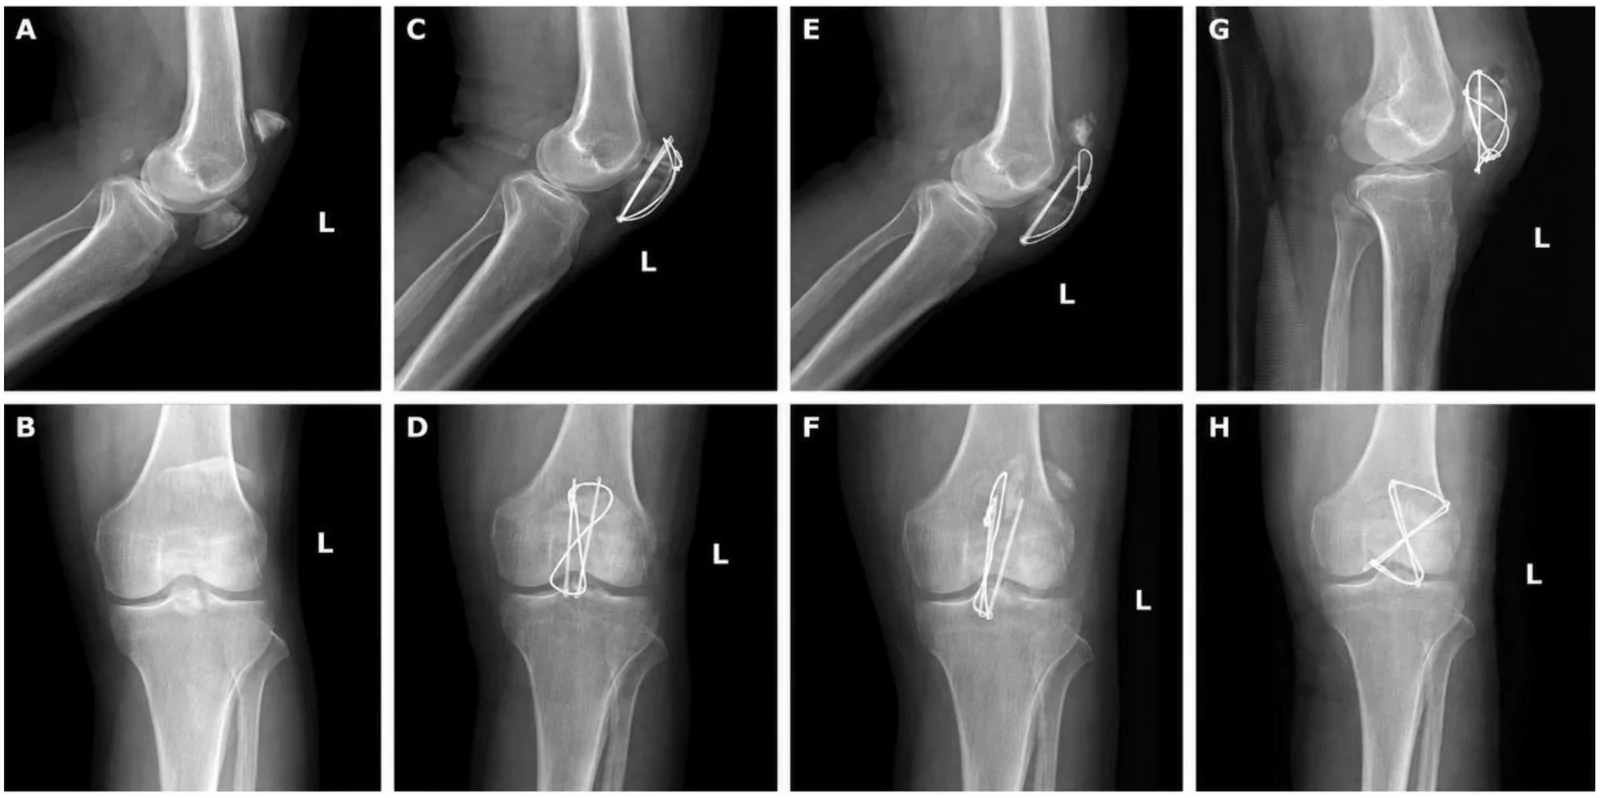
Radiographic evaluation during the clinical course. (A,B) Preoperative lateral and anteroposterior radiographs showing the initial left patellar fracture. (C,D) Immediate postoperative lateral and anteroposterior radiographs demonstrating satisfactory reduction and alignment after primary fixation. (E,F) Lateral and anteroposterior radiographs after the postoperative fall, showing secondary traumatic redisplacement with comminution involving the proximal pole of the patella. (G,H) Post-revision lateral and anteroposterior radiographs showing restored alignment and re-fixation stability.
